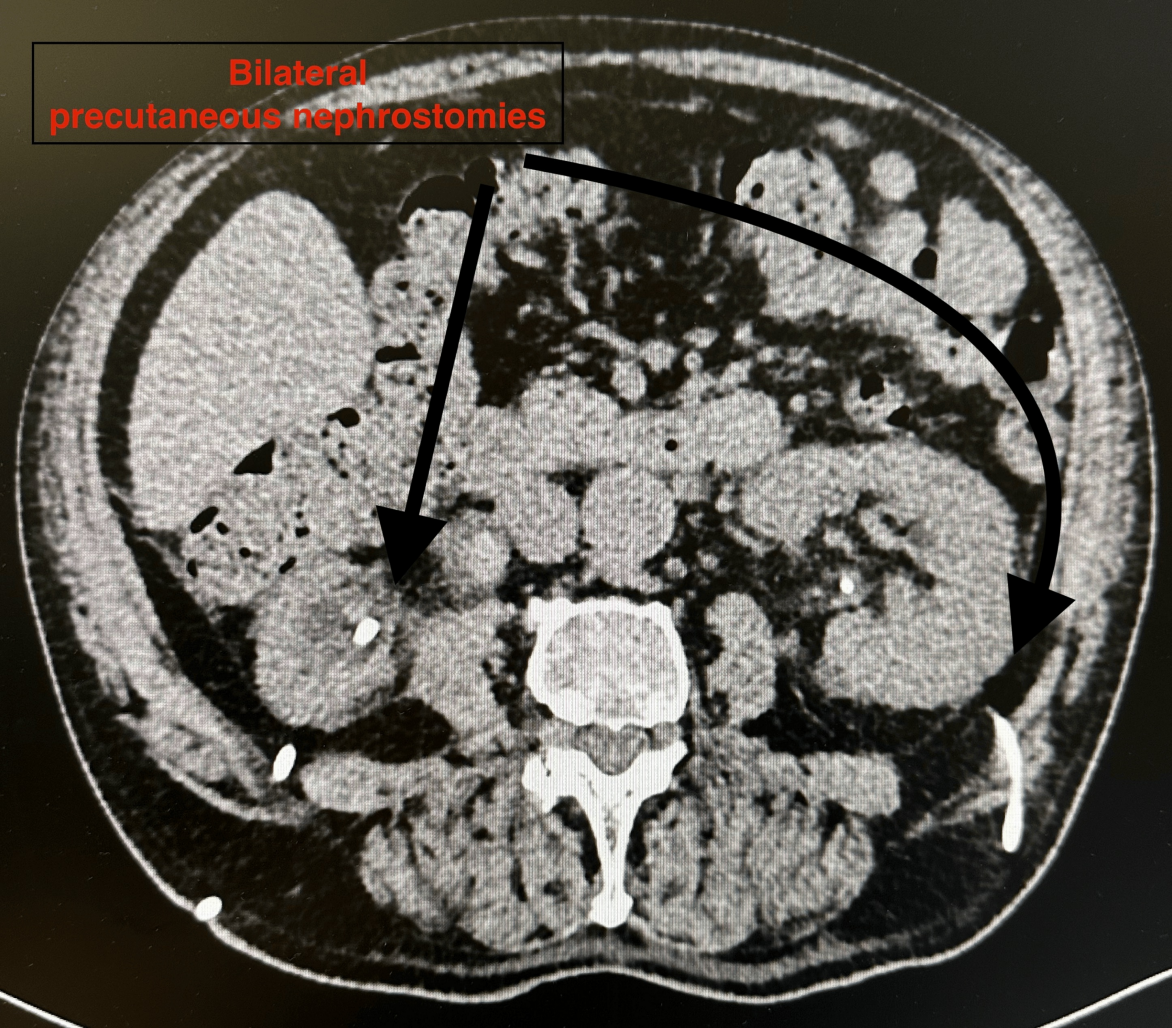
Axial CT image showing bilateral percutaneous nephrostomy tubes (black arrows) placed for relief of obstructive uropathy Moderate bilateral hydronephrosis is also visible CT: computed tomography
